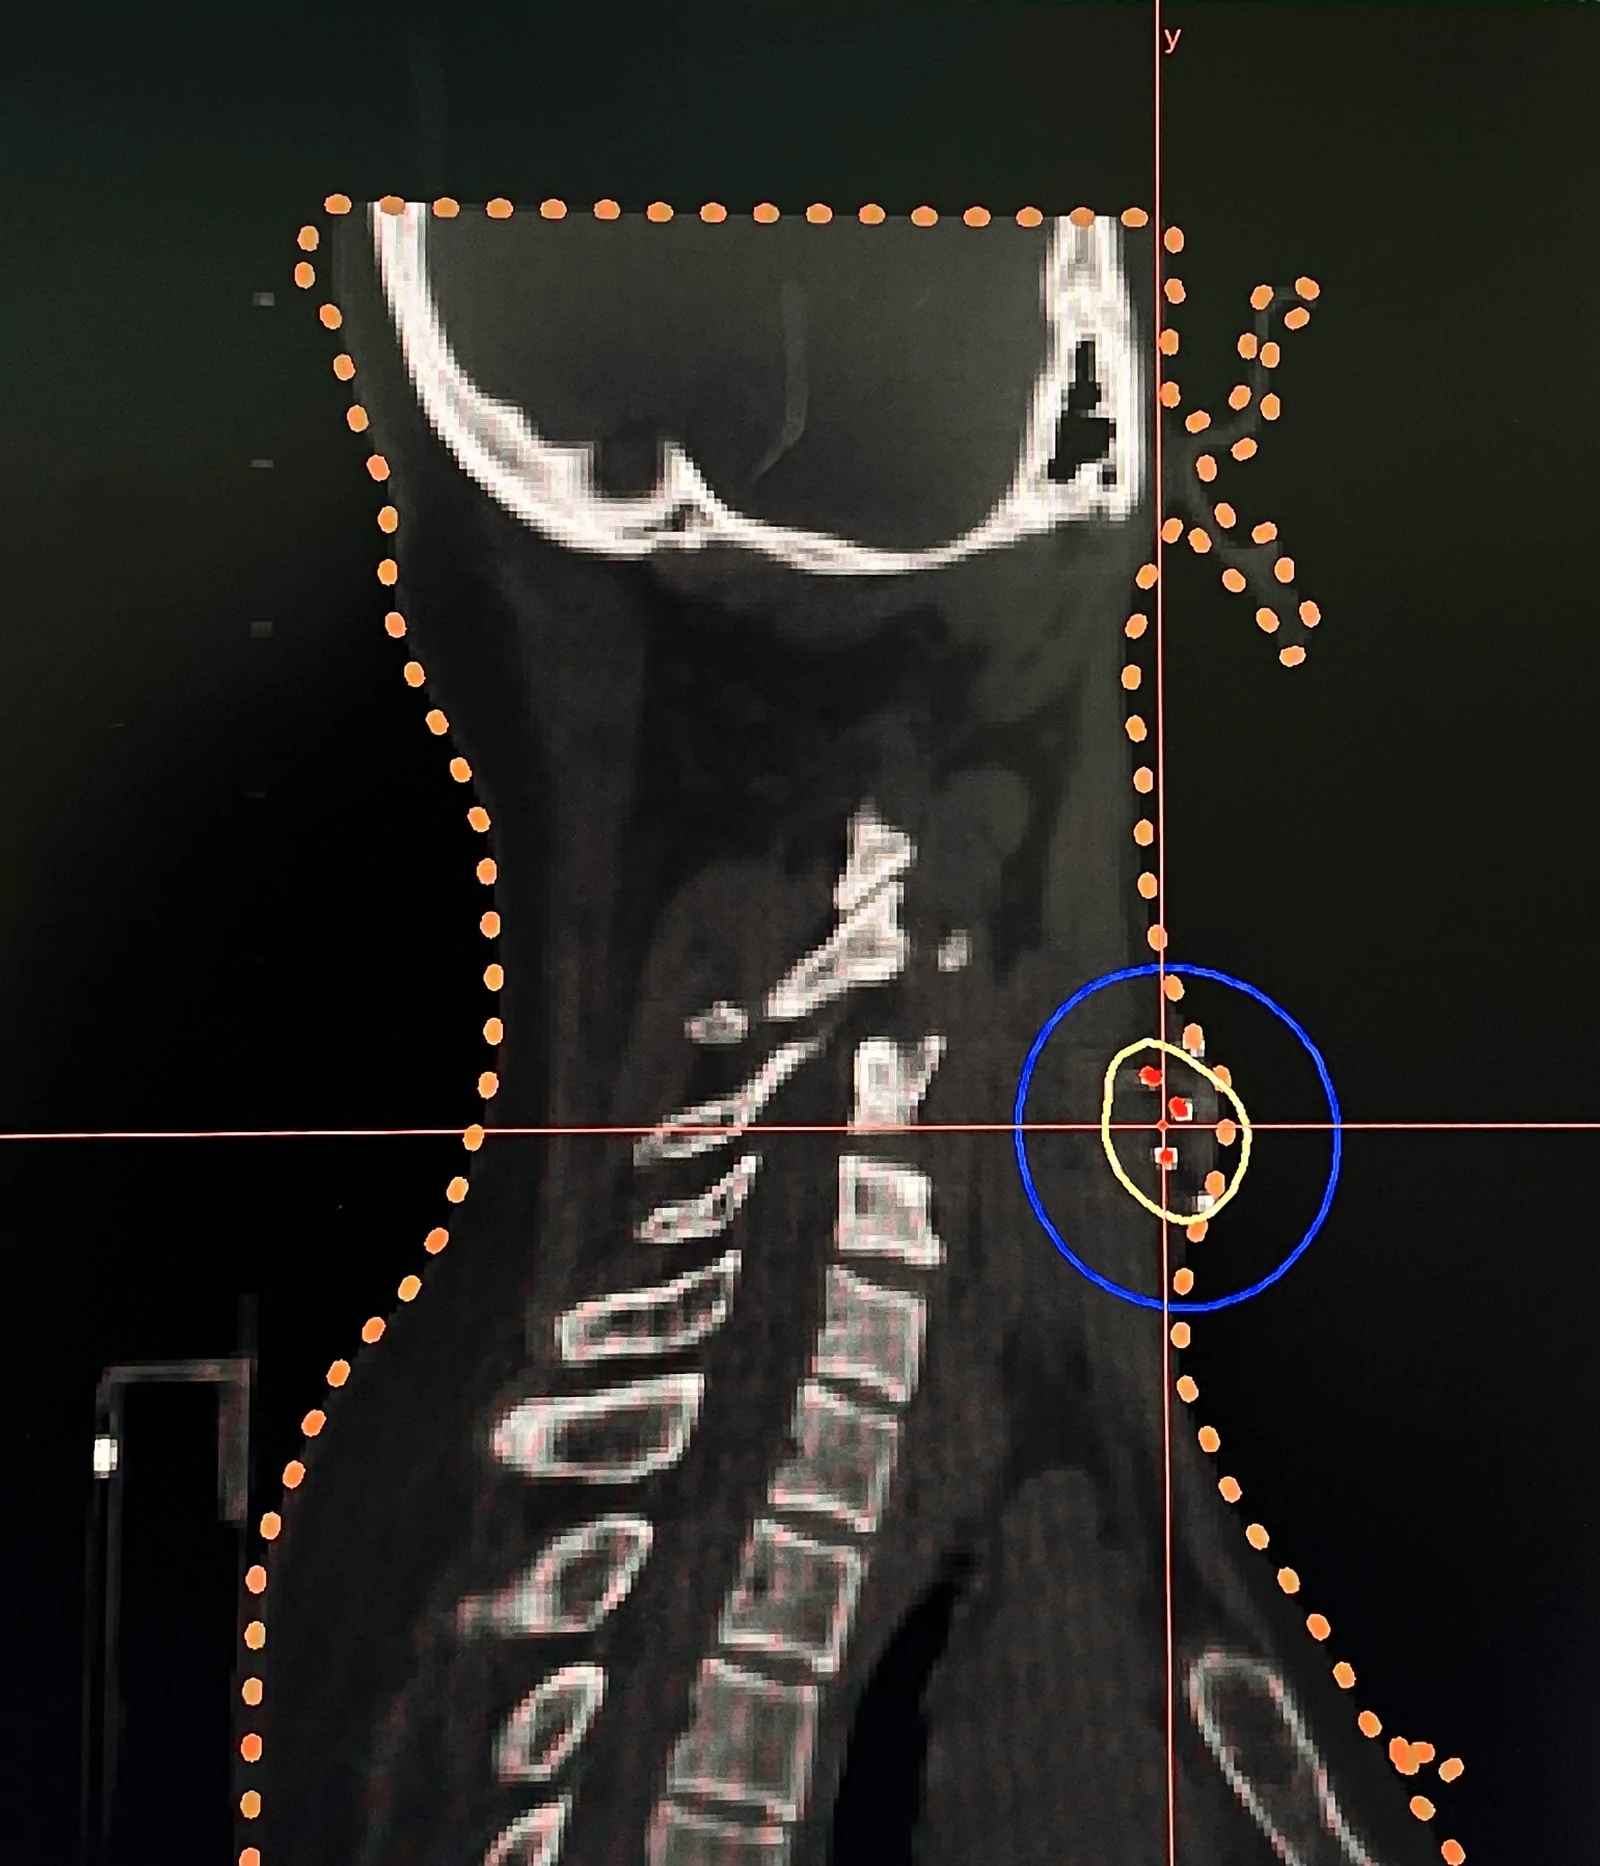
CT-based treatment plan showing catheter placement within the target lesion, with the 100% isodose line (yellow) closely conforming to the clinical target volume and the 30% isodose line (blue) demonstrating the steep dose fall-off sparing surrounding tissue.
